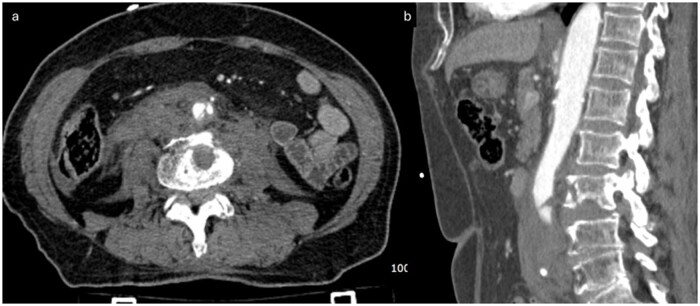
CT at admission in Emergency Department. (a) Axial arterial phase CT scan and (b) sagittal MPR reconstruction show complete lack of opacification of the abdominal aorta beginning at the level of L3. A fracture of the anterior vertebral body of L3 is clearly visible. In both planes, an intimal dissection flap is appreciable just proximal to the point of aortic occlusion, supporting the diagnosis of traumatic aortic dissection with subsequent intraluminal thrombosis.
